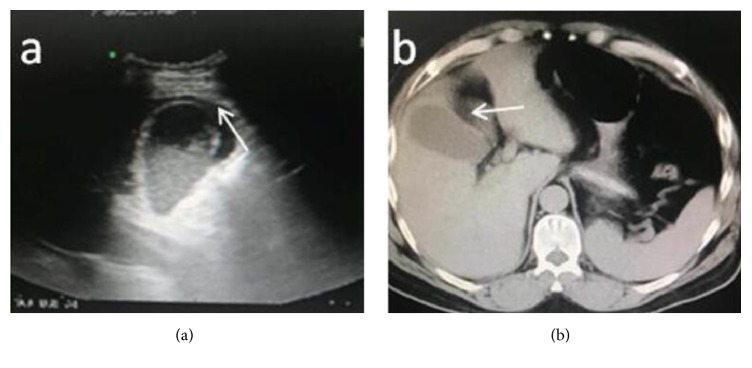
Preoperative abdominal ultrasonography and CT scan indicate pericholecystic collection (white arrow) in a patient on admission.
